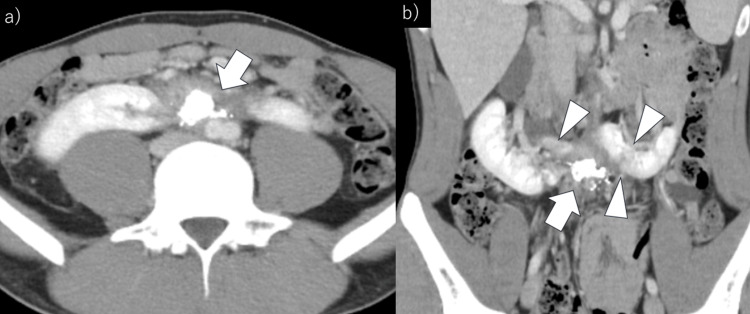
Three months after the procedure, follow-up contrast-enhanced computed tomography was performed. (a) Axial view: Further reduction of the retroperitoneal hematoma (arrow) is observed. (b) Coronal view: Retroperitoneal hematoma (arrow) is reduced, and the area of renal isthmus infarction (arrowheads) is decreased.
